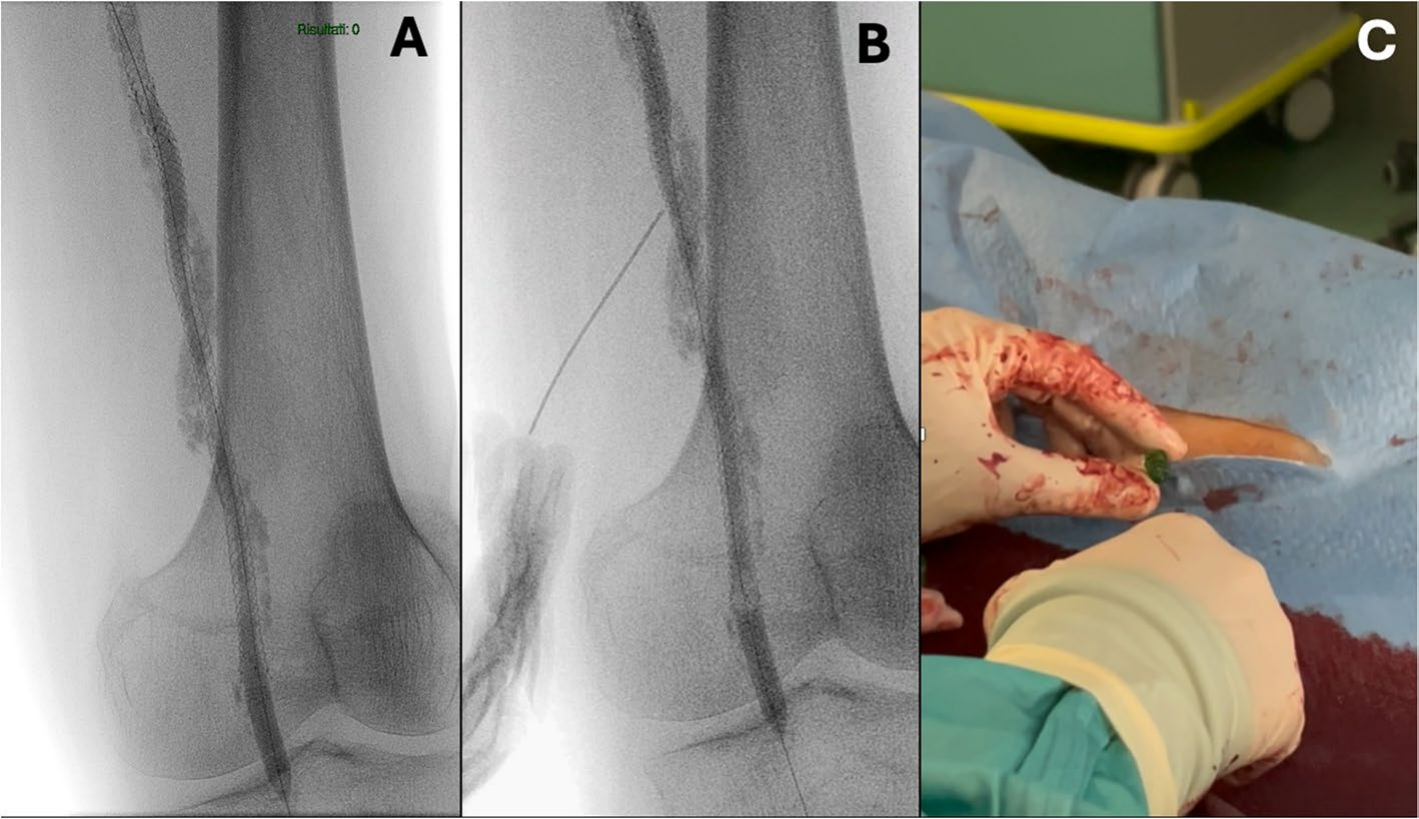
During stents post-dilation, upon completion of the inflation phase, the balloon failed to deflate despite repeated attempts using standard techniques. A The balloon catheter results in being undeflatable. B Direct puncture of the balloon catheter through the stent’s struts is performed under X-ray guidance. C: back “bleeding” (saline and contrast medium) is observed. Aspiration through the needle successfully facilitated the deflation of the balloon catheter
